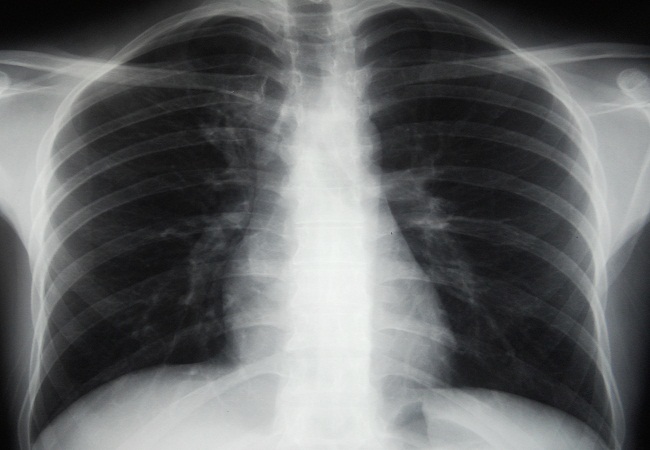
La radiographie thoracique de contrôle à la fin du traitement (6éme mois) objective une régression complète des lésions radiologiques
